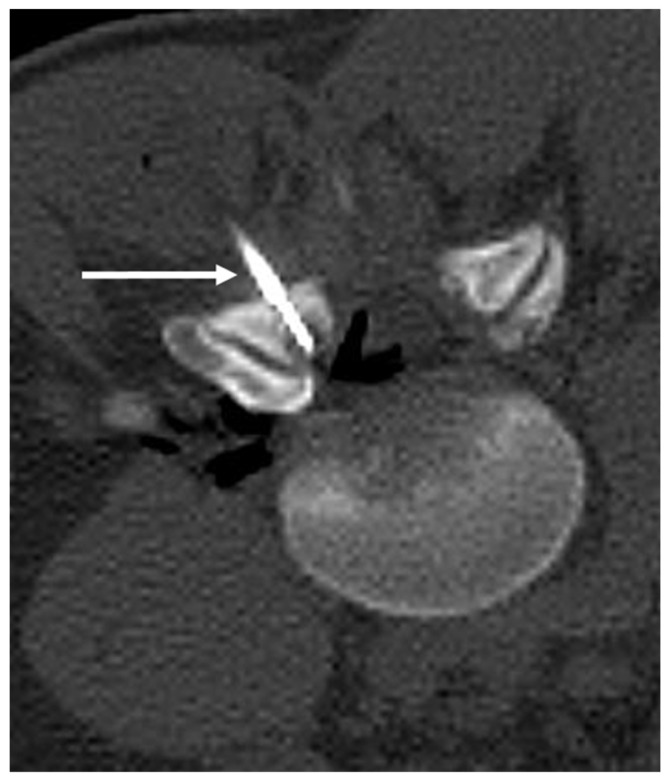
Axial CT image obtained during ablation of the osteoid shown in Figure 3 and Figure 4. Note the electrode placement (white arrow) with tip within the nidus. Air has been injected into the epidural space for neuroprotection (black).
